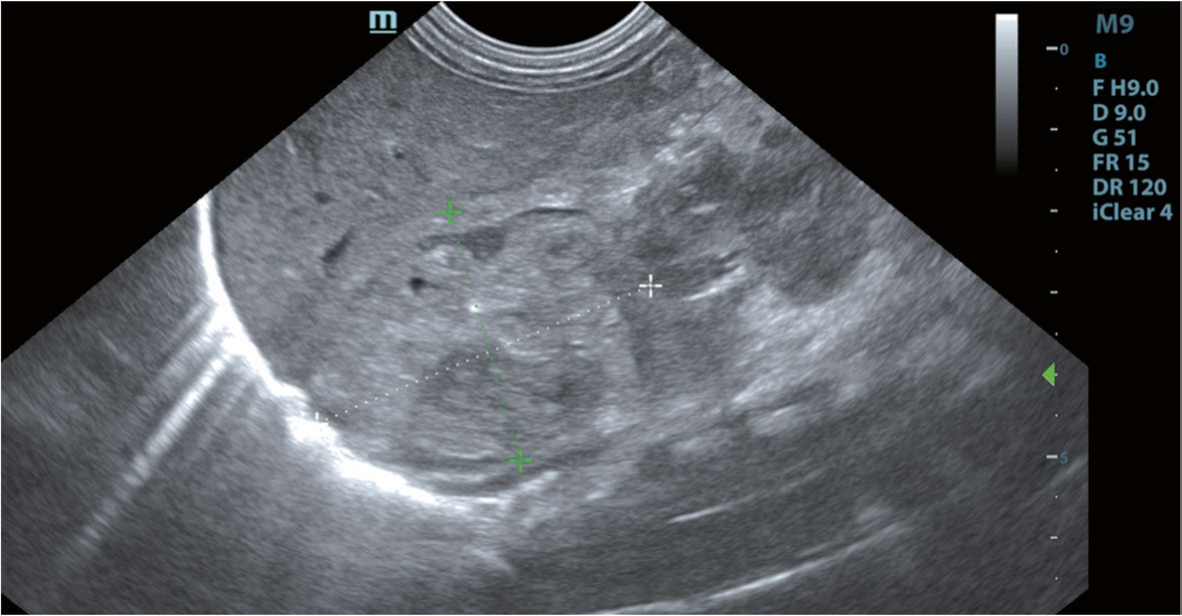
Abdominal ultrasound showed a huge mass in the right adrenal gland
